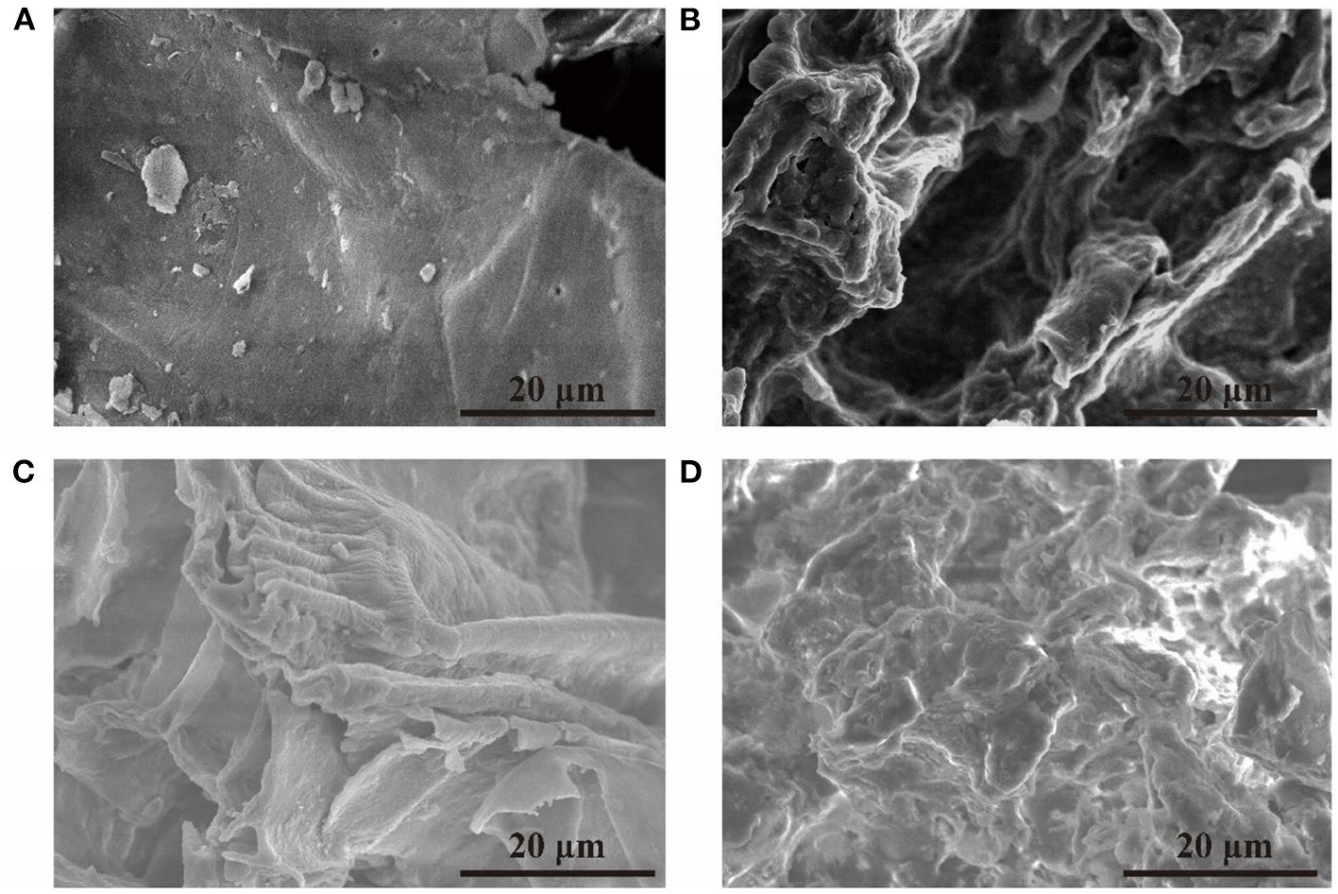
The SEM images of sugarcane bagasse with different pretreatments. (A) Without pretreatment, (B) with ultrasonic-assisted single alkali pretreatment, (C) with ultrasonic-assisted mixed alkali pretreatment, (D) with ultrasonic–assisted mixed alkali pretreatment and enzymatic hydrolysis.
